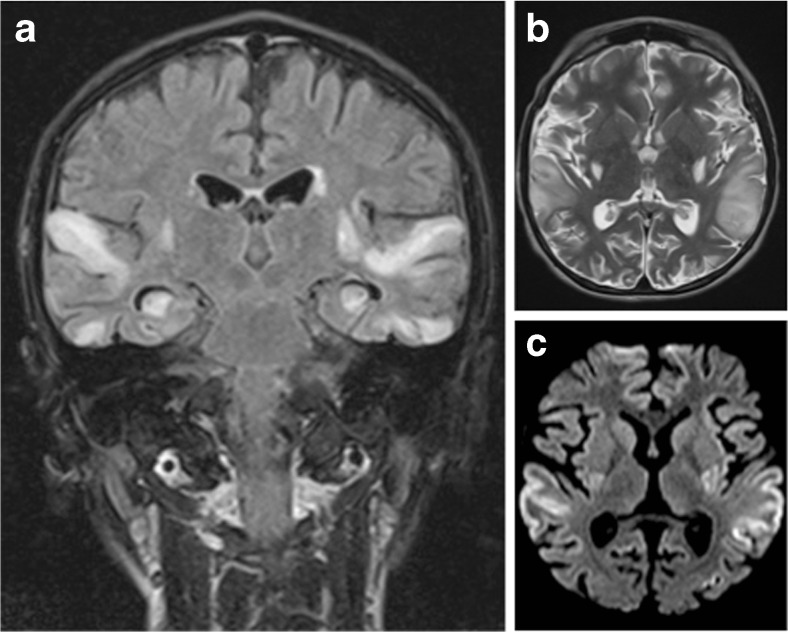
Brain MRI scan 72 h after onset of symptoms. a Coronal FLAIR image shows symmetrical high signal intensities lesions in multiple arterial territories. b Axial T2-weighted image demonstrating high signal cortical and subcortical lesions bilaterally in the edematous superior temporal gyri. c Axial diffusion-weighted image (DWI) demonstrating high signal areas in the same regions
